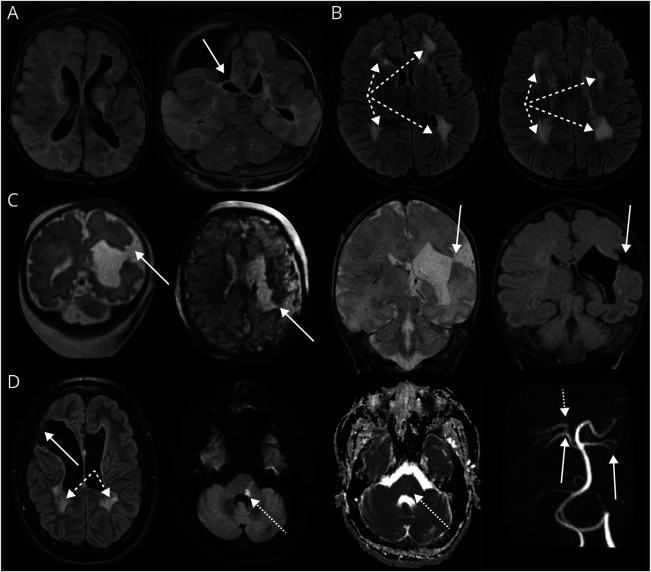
Neuroimaging Patterns in *COL4A1*-Related Disorder (A) PVHI pattern with porencephalic cysts (thick white arrow) in a patient with *COL4A1* variant with perinatal presentation (axial and coronal T2/FLAIR), (B) SVID pattern (dashed thick arrows) in patient with *COL4A1* variant with late childhood presentation (axial T2/FLAIR), (C) PVHI pattern (thick white arrows) in patient with *COL4A1* variant, fetal MRI (coronal HASTE and axial EPI SWI), and postnatal MRI (coronal T2WI and T1WI), (D) PVHI pattern (thick white arrows) on SVID background (dashed thick arrows) in a patient with *COL4A1* variant with perinatal presentation despite diagnosis being delayed until late adolescence secondary to a subacute left pontine stroke shown on DWI/ADC (dotted thick arrows). Vessel imaging demonstrates tortuosity of posterior circulation, particularly involving the vertebrobasilar system with dominant left vertebral artery and irregularity of right posterior cerebral (thin dotted arrow) and bilateral superior cerebellar arteries (thin white arrows) on MR angiogram. Each panel represents a different patient. ADC = apparent diffusion coefficient; DWI = diffusion-weighted imaging; EPI SWI = echo planar imaging susceptibility weighted imaging; FLAIR = fluid-attenuated inversion recovery; HASTE = half Fourier acquisition single-shot turbo spin echo; PVHI = periventricular hemorrhagic infarction; SVID = small vessel ischemic disease; T1WI = T1-weighted imaging; T2WI = T2-weighted imaging.
